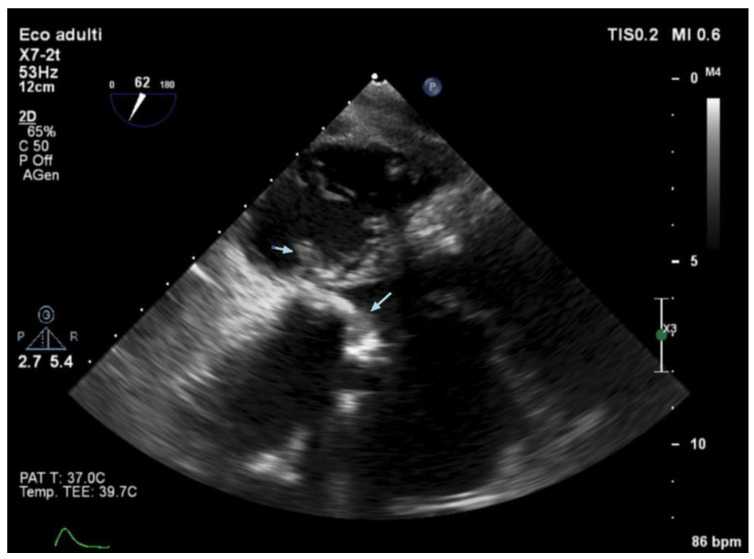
Transesophageal echocardiography—deep transgastric 5-chamber view. Tricuspid valve (left of image) showing a rounded, isohyporeflecting vegetative lesion (arrow) located between the septal leaflet and the anterior leaflet of the valve. Aortic bioprosthesis (right of image) showing diffuse, irregular leaflet thickening (arrow) due to the presence of mobile, endocarditic vegetative lesions.
